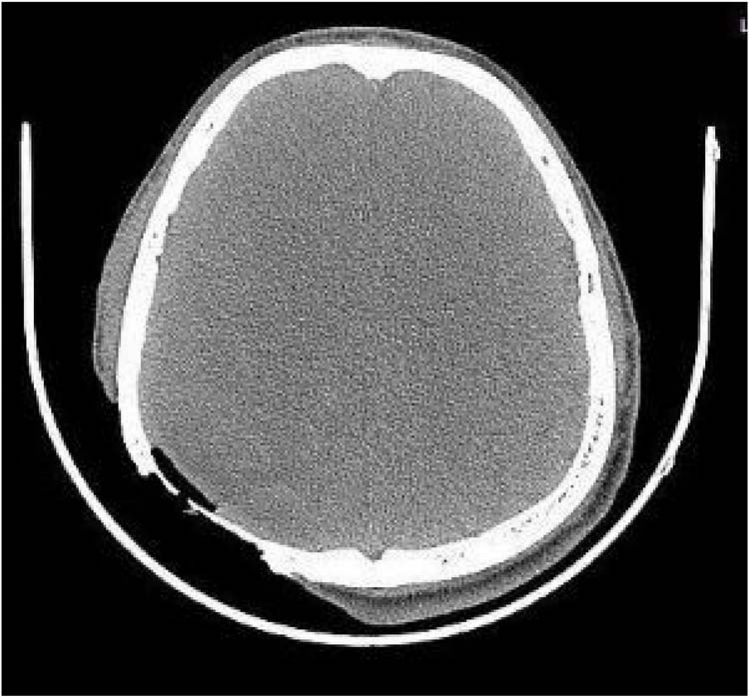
CT shows the presence of gas inside the skull.
